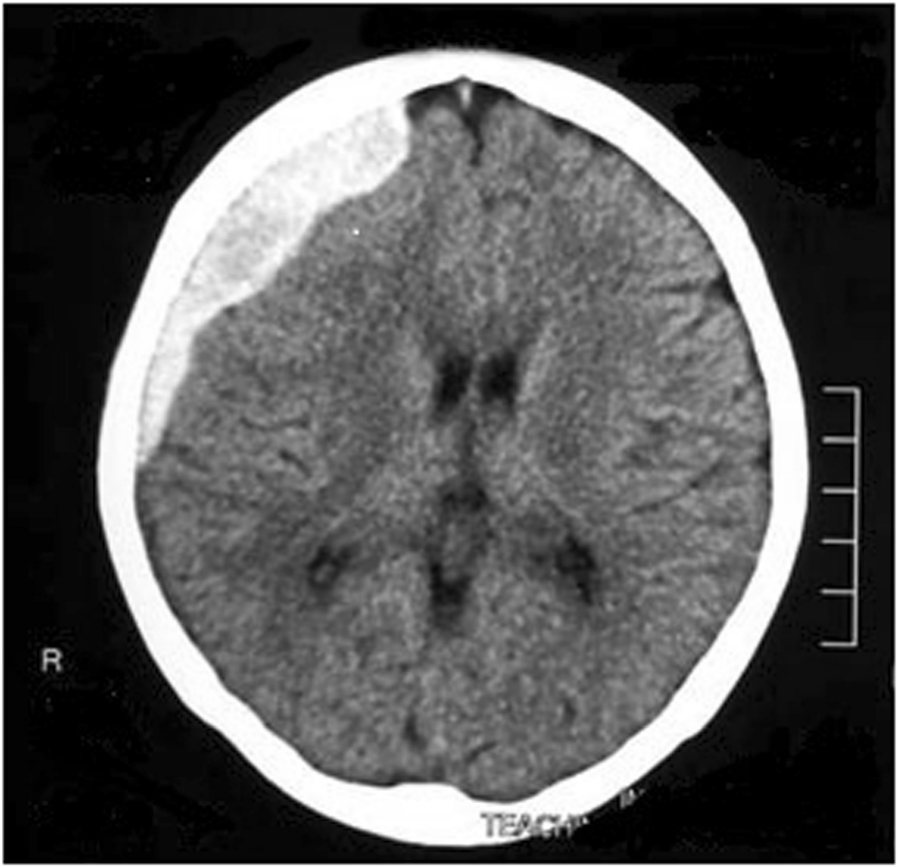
Image of computed tomography scan of brain on postpartum day 23: this shows a right-sided fronto-parietal subdural haemorrhage with midline shift.
